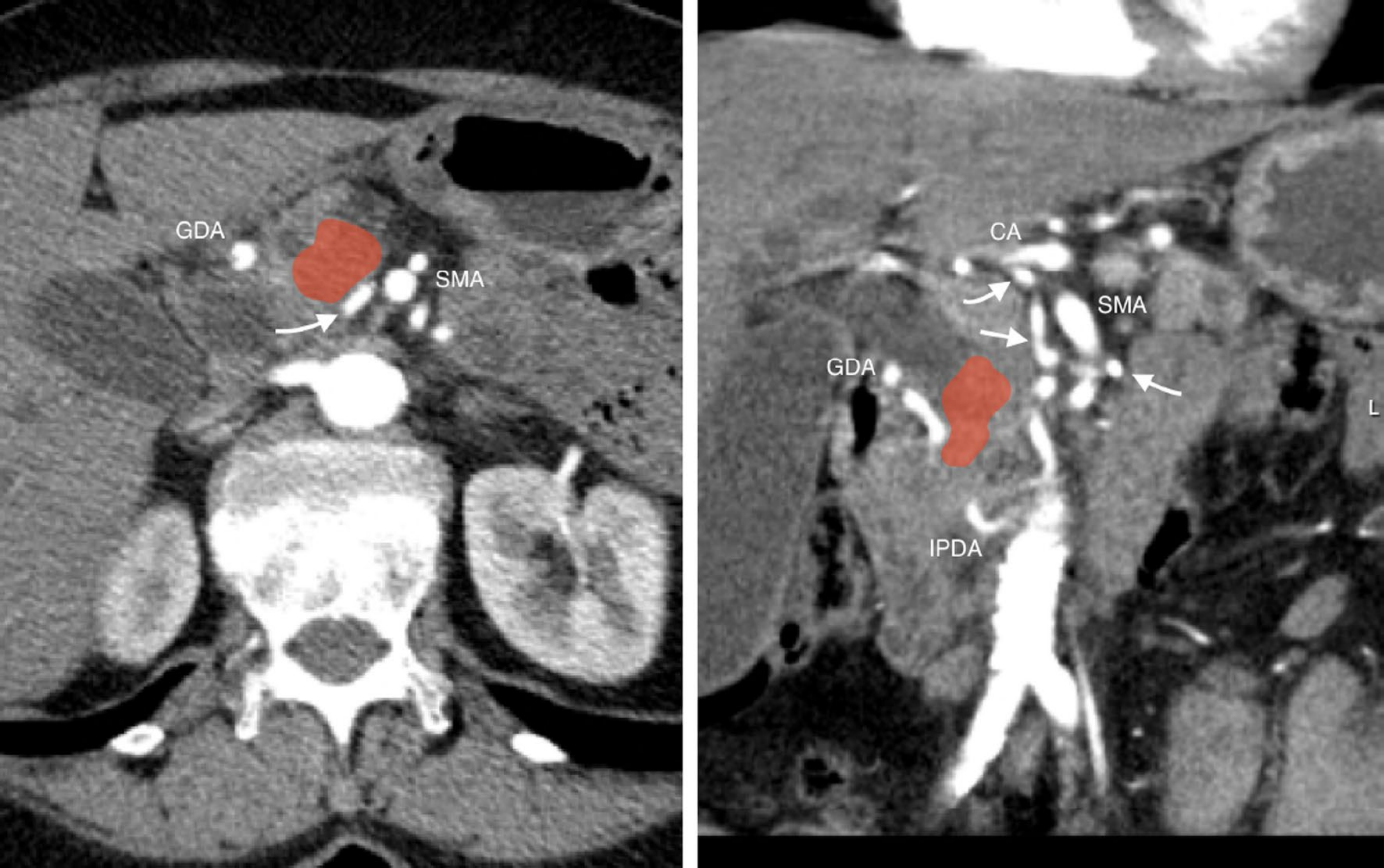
Computed tomography image showing the tumor of the pancreatic head (*highlighted red*) in proximity with the the arc of Buhler (*white arrowheads*) and the *GDA* gastroduodenal artery. *SMA* superior mesenteric artery, *CA* celiac artery, *PDA* inferior pancreaticoduodenal arteries
